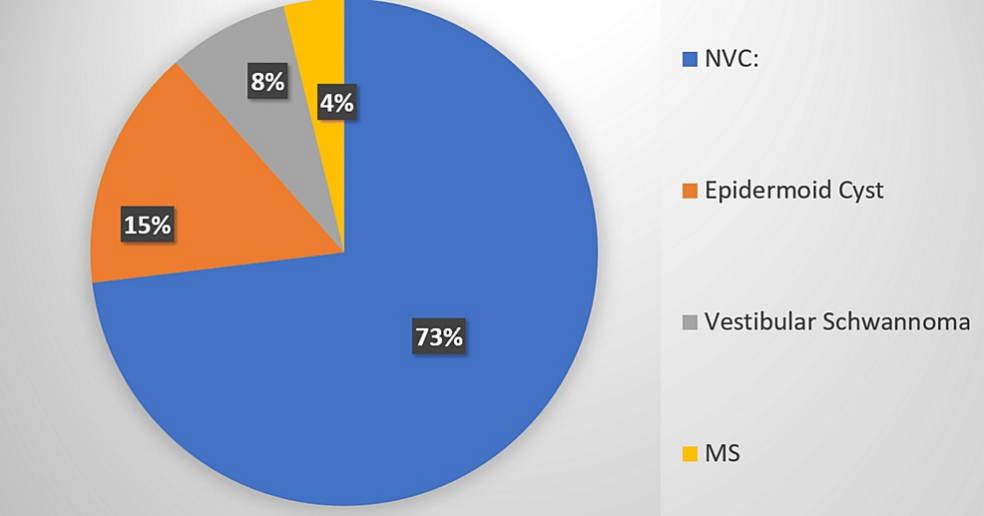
Etiology obtained on MRI NVC: Neurovascular compression MS: Multiple sclerosis
